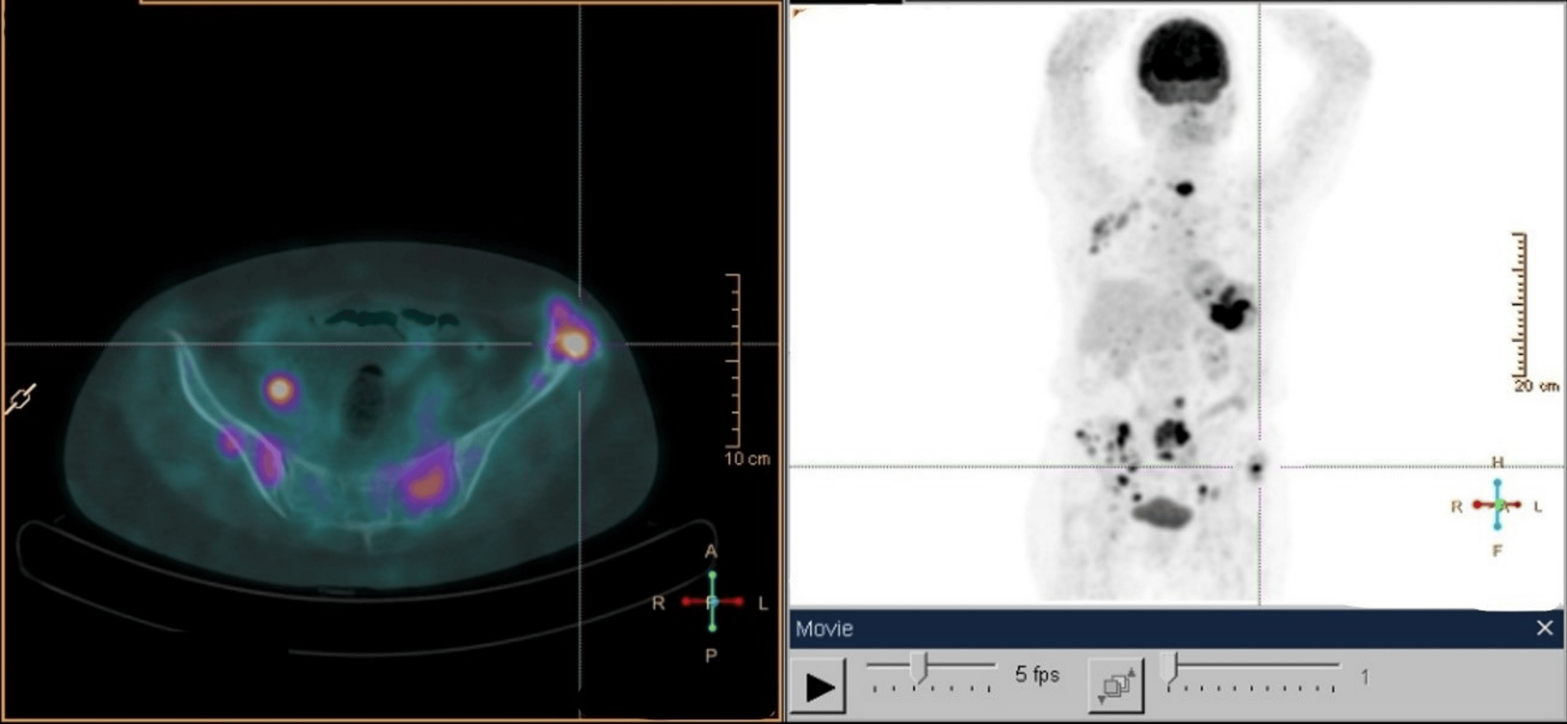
18F-FDG PET-CT PET-CT suggesting malignant neoplastic involvement in the supra- and infra-diaphragmatic lymph nodes, splenic, and bone (multiple lesions involving pelvic bones, multiple vertebral elements, the sternum, and the right seventh costal arch). with possible involvement of the gastric fundus 18F-FDG PET-CT: 18F-fluorodeoxyglucose positron emission tomography-computed tomography
